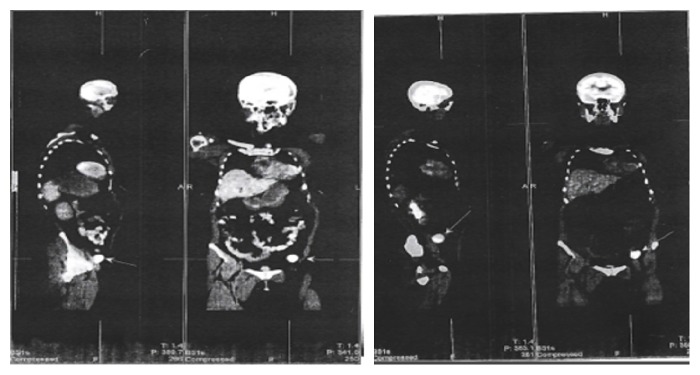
PET-CT images of the patient taken six months apart after recurrence. The place shown with the arrow is overmassed.
